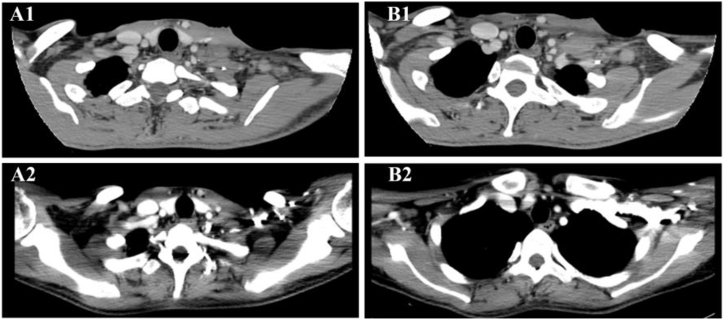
Comparison of image findings pre-and post-treatment. (a1, b1) The neck CT on July 2023, indicated enlarged lymph nodes in the neck. (a2, b2) After antimycobacterial treatment, the neck CT on November 2023, showed some of the lymph nodes shrank.
